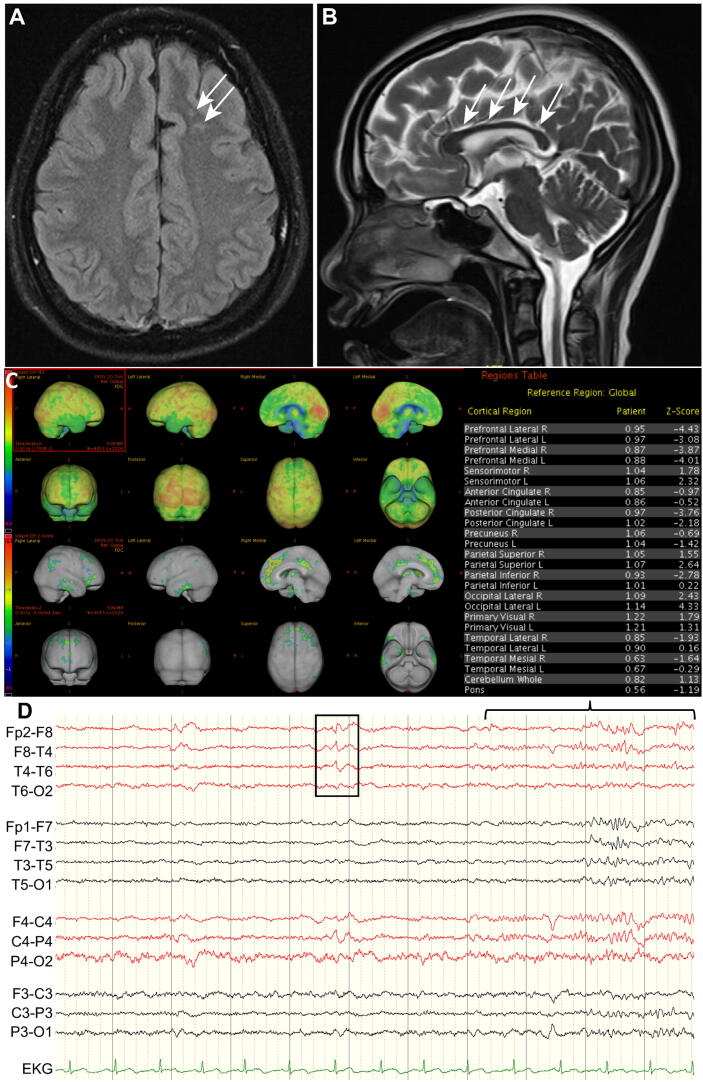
Neuroimages and video-electroencephalographic findings of the patient. (A) Axial view of a scan obtained through brain magnetic resonance imaging (MRI) fluid-attenuated inversion recovery showing several nodules at the left frontal centrum semiovale with a signal intensity similar to that of the gray matter (arrows); the largest one was approximately 10 mm in diameter. (B) MRI coronal view revealing a relatively thin corpus callosum (arrows). (C) Three-dimensional demonstration of brain ^18^F-fluorodeoxyglucose positron emission tomography image with quantification parameters indicating reduced metabolism in the bilateral frontal lobes, right parietotemporal lobes, and the bilateral posterior cingulate but with more reduction on the right side. (D) Video-electroencephalography showing focal sharp waves and spike-and-wave complexes with phase reversals at F8 (square frame), followed by fast activities originating from the right hemisphere after a few seconds (bracket).
